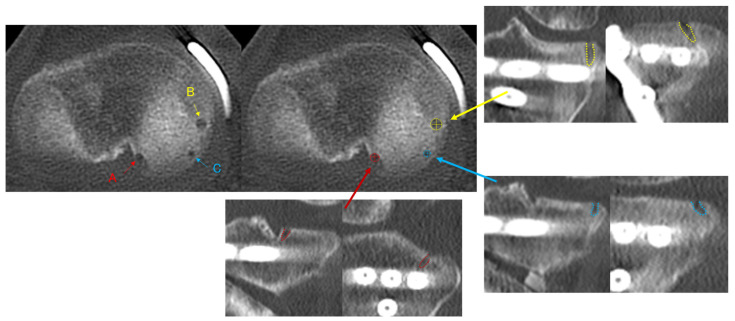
CT images showing anchor holes and anchor hole width (AHW) for each anchor: (A) the anchor hole for the anchor used in MMPRT repair (R-anchor), (B) the anchor hole for the midbody anchor (M-anchor) used in the centralization technique, and (C) the anchor hole for the midbody–posterior anchor (MP-anchor). The maximum anteroposterior and mediolateral AHW (AP-AHW and ML-AHW) were measured.
